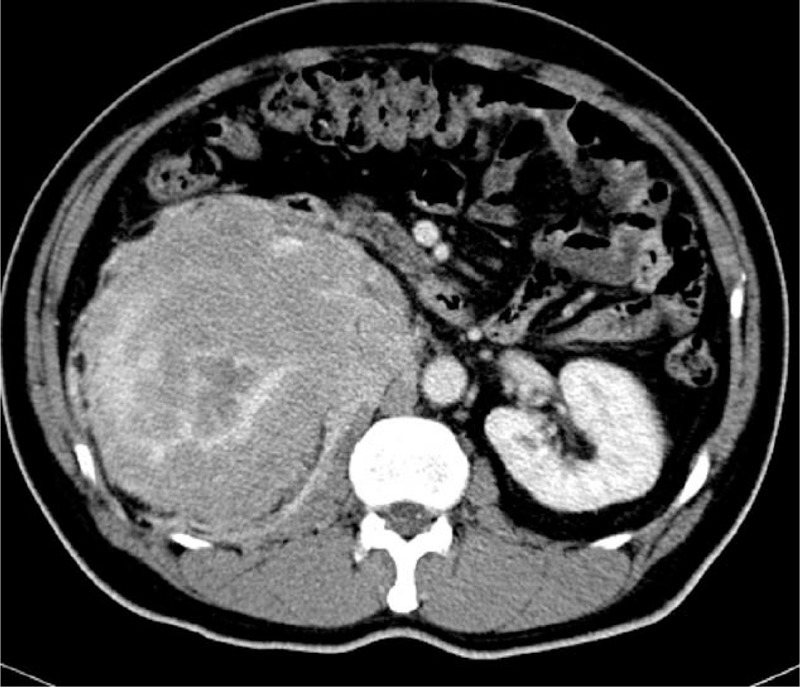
Contrast enhanced computed tomography scan revealing a large heterogeneously enhancing mass involving the right kidney.
